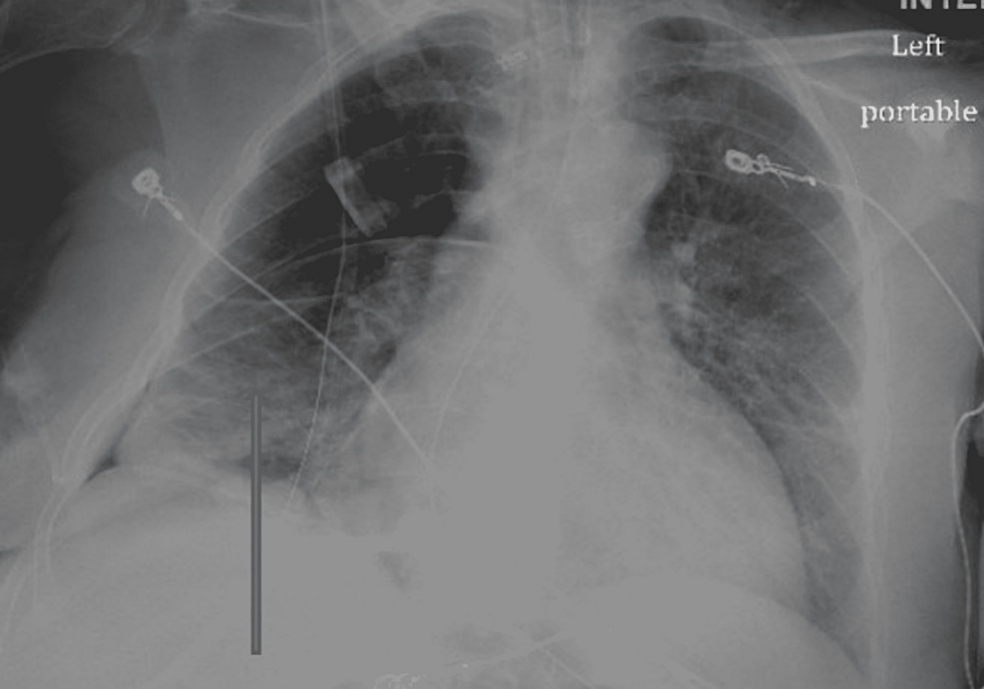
Portable chest X-ray anteroposterior view showing significant improvement in the right hemithorax (arrow).
